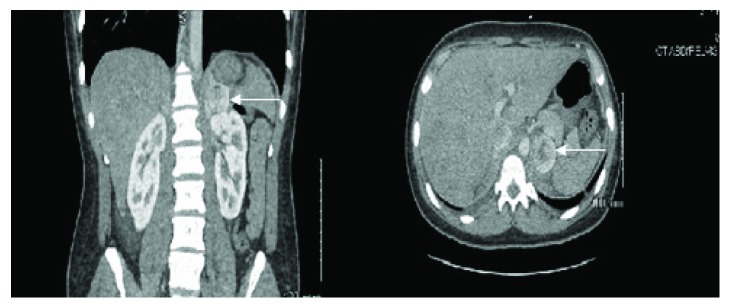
Abdomen/pelvic CT with contrast, coronal, and axial views showing the 4 × 3 cm left pheochromocytoma.
